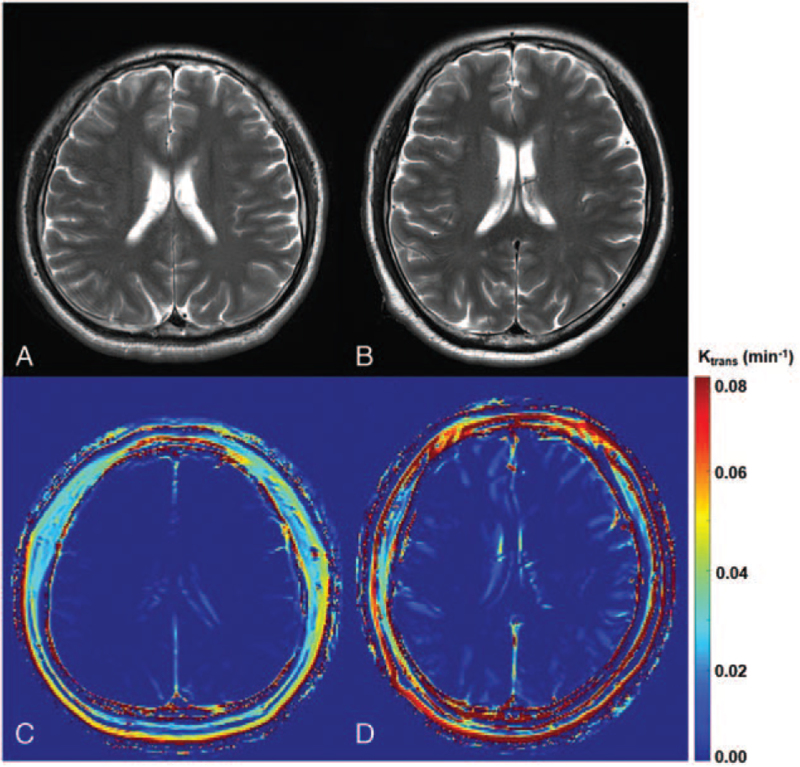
T2-weighted magnetic resonance imaging (MRI) and K_trans_ map of dynamic contrast-enhanced MRI of the healthy control participant (the 42-year-old man) and the patient (the 45-year-old man). (A) The T2-weighted MRI of the healthy control participant and (B) that of the patient show no abnormal finding. Ten round regions of interest (5 on each hemisphere) are depicted on the K_trans_ map of dynamic contrast-enhanced (DCE)-MRI of the healthy control participant and the patient (dotted circles in Figure c). (C) K_trans_ map of DCE-MRI of the healthy control participant shows no abnormal finding; however, (D) K_trans_ map of DCE-MRI of the patients shows bright blue colored lines throughout the overall sulcus.
